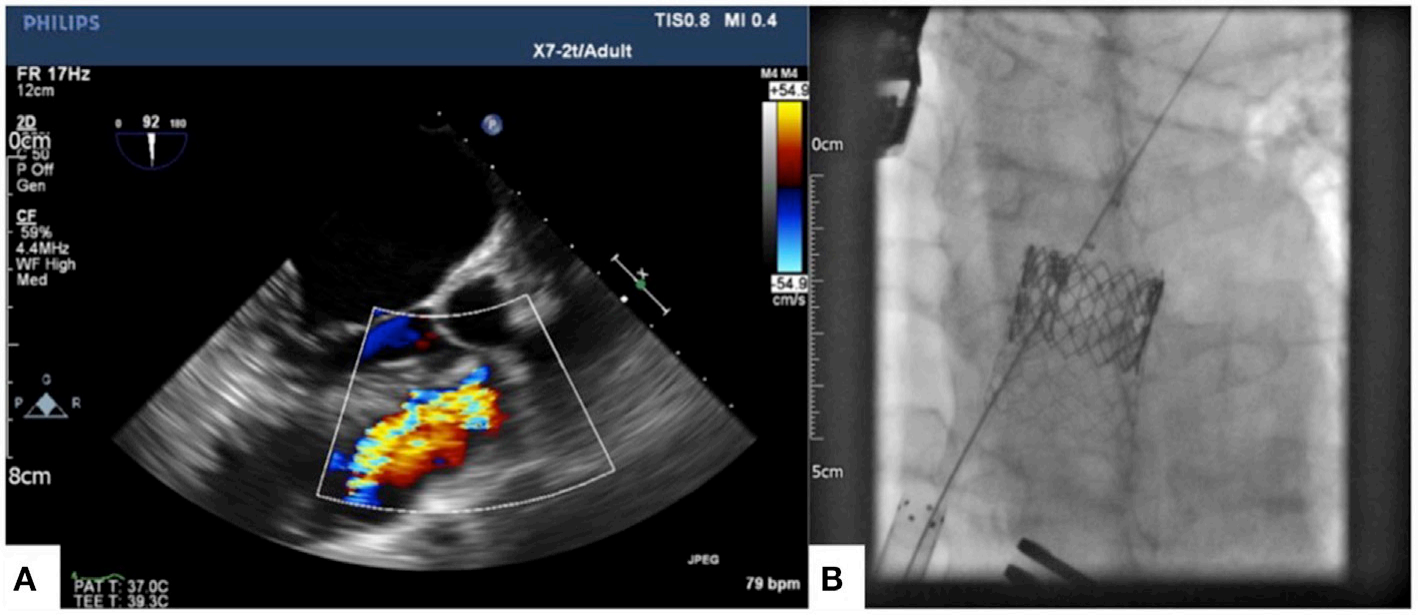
Baseline pulmonary regurgitation showed by intraoperative transesophageal echocardiography (A) and 29-mm Edwards Sapien XT prosthesis within an Andramed AS43XXL stent implanted in the pulmonary position (B).
